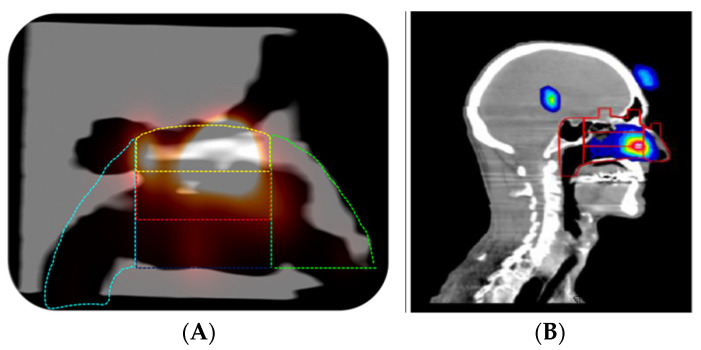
In vitro (A) vs. in vivo (B) deposition patterns as assessed by scintigraphy. The colour lines in the figure outline the regions of interest (ROI) in both the nasal cast (A) and human volunteers (B).
